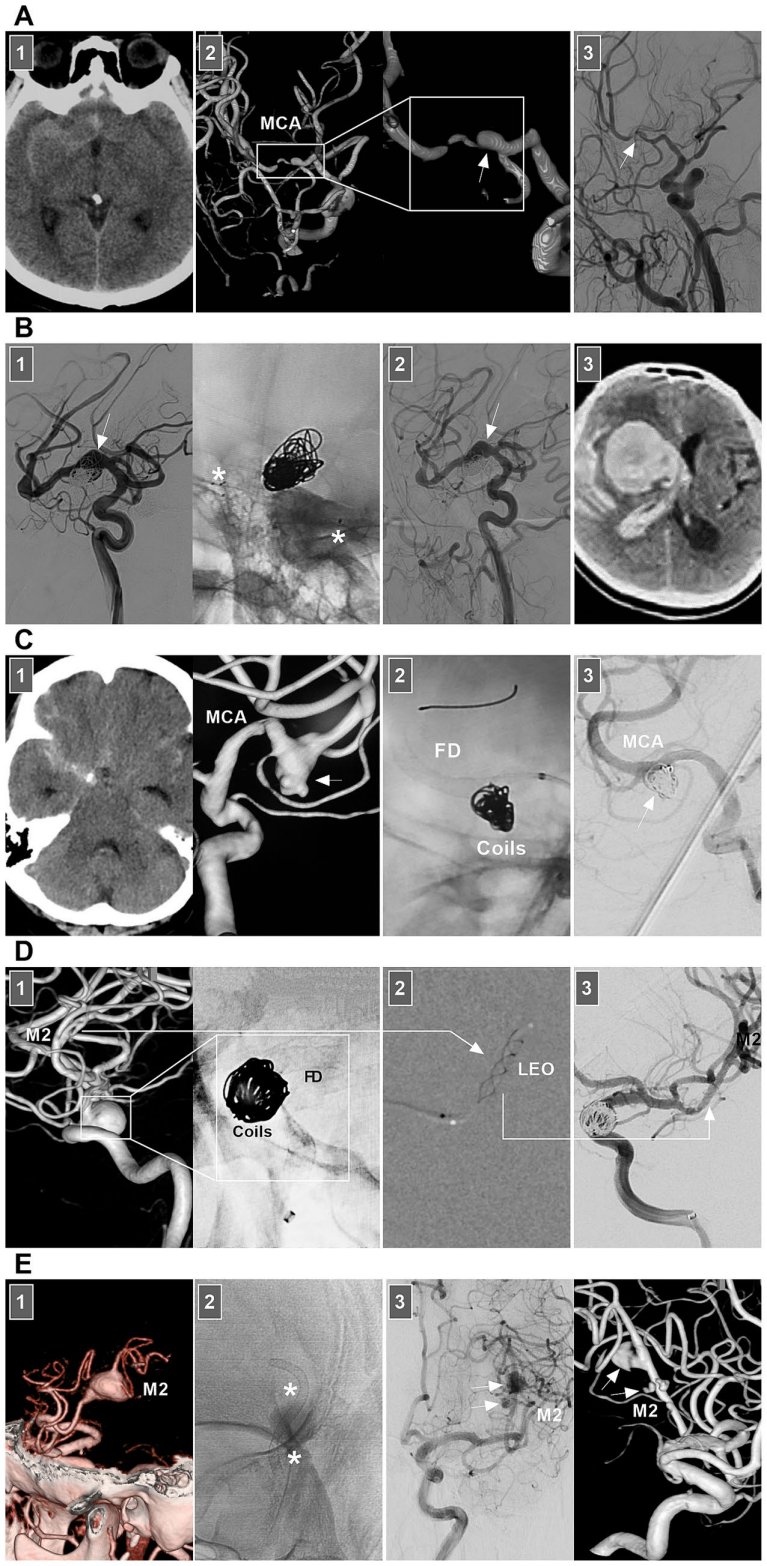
Treatment of MCA dissections. (A) Panel 1: CT showing SAH. Panel 2: DSA images showing a dissection (frames) at MCA trunk with stenosis and dilatation (arrow). Panel 3: Three-month follow-up DSA showing that the dissection (arrow) regressed. (B) Panel 1: DSA (left panel) and X-ray image (right panel) showing a fusiform MCA dissection (arrow) was coiled under the assistance of traditional stenting (asterisks). Panel 2: One-year follow-up DSA showing that the dissecting aneurysm (arrow) was stable and did not grow. Panel 3: Three-year follow-up CT showing fatal intracranial hemorrhage, indicating the dissection ruptured. (C) Panel 1: CT (left panel) showing SAH, DSA (right panel) showing an MCA dissecting aneurysm (arrow). Panel 2: X-ray image showing the FD-assisted coiling. Panel 3: DSA showing that the dissecting aneurysm (arrow) was embolized. (D) Panel 1: DSA (left panel) and X-ray image (right panel) showing a supraclinoid ICA aneurysm was coiled by the assistance of FD deployment (frames), there was a M2 dissection. Panel 2: Roadmap image showing the M2 dissection was stented by LEO baby (long arrow). Panel 3: DSA showing the reconstructed M2 (long arrow). (E) Panel 1: CT angiography showing a M2 dissection. Panel 2: X-ray image showing the dissection was stented by FD deployment (asterisks). Panel 3: DSA (left panel) and its reconstructive image (right panel) of six-month follow-up showing that the M2 segment was reconstructed with less residual dissection (arrows). CT, computed tomography; DSA, digital subtraction angiography; EVT, endovascular treatment; FD, flow diverter; MCA, middle cerebral artery; M2, second segment of MCA; SAH, subarachnoid hemorrhage.
